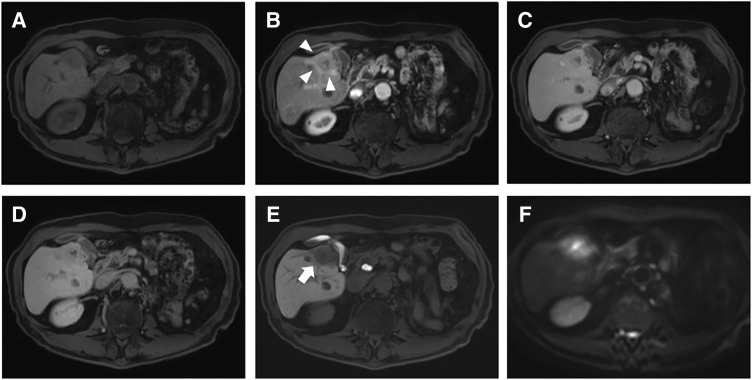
(A) The unenhanced image shows a 38-mm hypointense lesion in segment 5 of the liver. (B) Arterial-phase MRI demonstrates peritumoral enhancement (white arrowheads) with multifocal necrotic areas in the lesion. (C) Portal venous-phase image shows faint progressive internal enhancement in the lesion. (D) Transitional-phase image shows faint enhancement in the lesion. (E) During the 20-min hepatobiliary phase after contrast administration, a peritumoral hypointense area is observed (white arrow). (F) DWI shows abnormal signal intensity of the lesion. DWI, diffusion-weighted imaging
